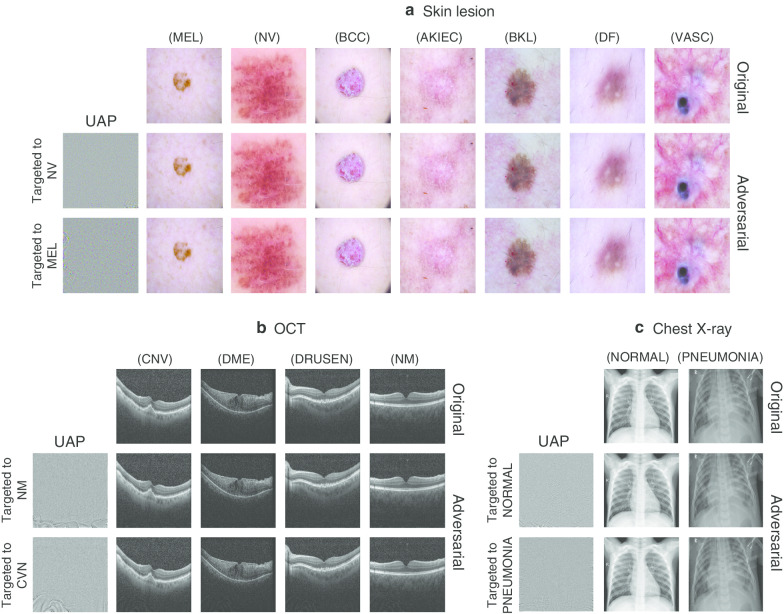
Targeted UAPs with $$p = 2$$ against Inception V3 models and their adversarial images for skin lesions (a), OCT (b), and chest X-ray image datasets. Further, $$\zeta = 2\%$$ in a and c. $$\zeta = 6\%$$ in b. Labels in brackets beside the images are predicted classes. Original (clean) images were correctly classified into their actual labels. Adversarial images were classified into the target classes. UAPs are emphatically displayed for clarity; in particular, each UAP is scaled by a maximum of 1 and minimum of 0
